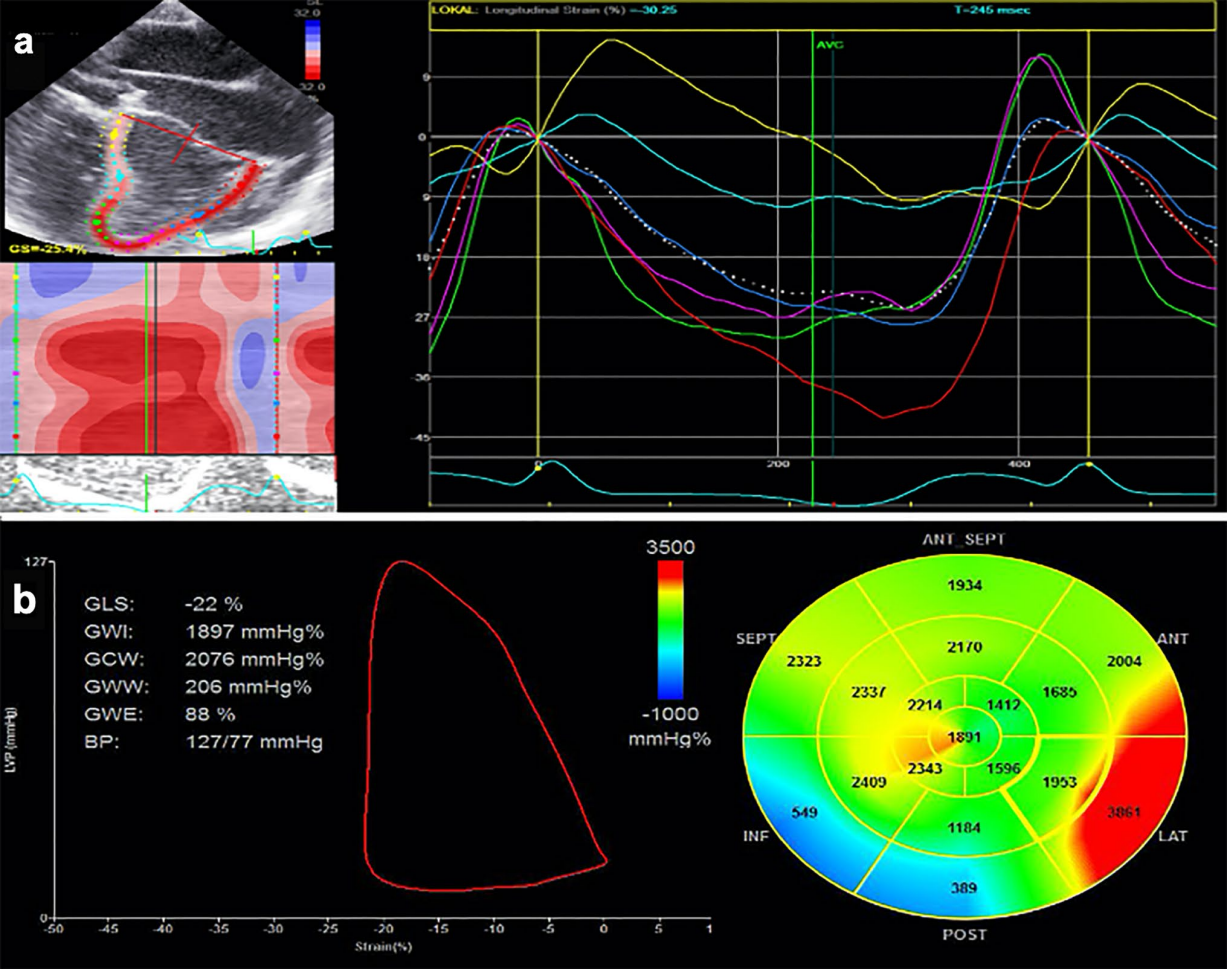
Exemplary demonstration of speckle trasssscking and myocardial work analysis. a Analysis of left ventricular peak systolic longitudinal strain obtained from a modified apical 4-chamber view with parametric (color-coded) display of end-systolic strain (left upper panel); M-mode representation of peak systolic strain (left lower panel); and strain-time curves: each colored curve displays a different segmental strain while the dotted white curve represents the average peak systolic longitudinal strain (right upper panel). b myocardial work indices obtained from pressure-strain loops with the myocardial work curve and the Bull’s eye representing segmental myocardial work indices. *GLS* global longitudinal strain, *GWI* global myocardial work index, *GCW* global constructive work, *GWW* global wasted work, *GWE* global effective work, *BP* blood pressure
